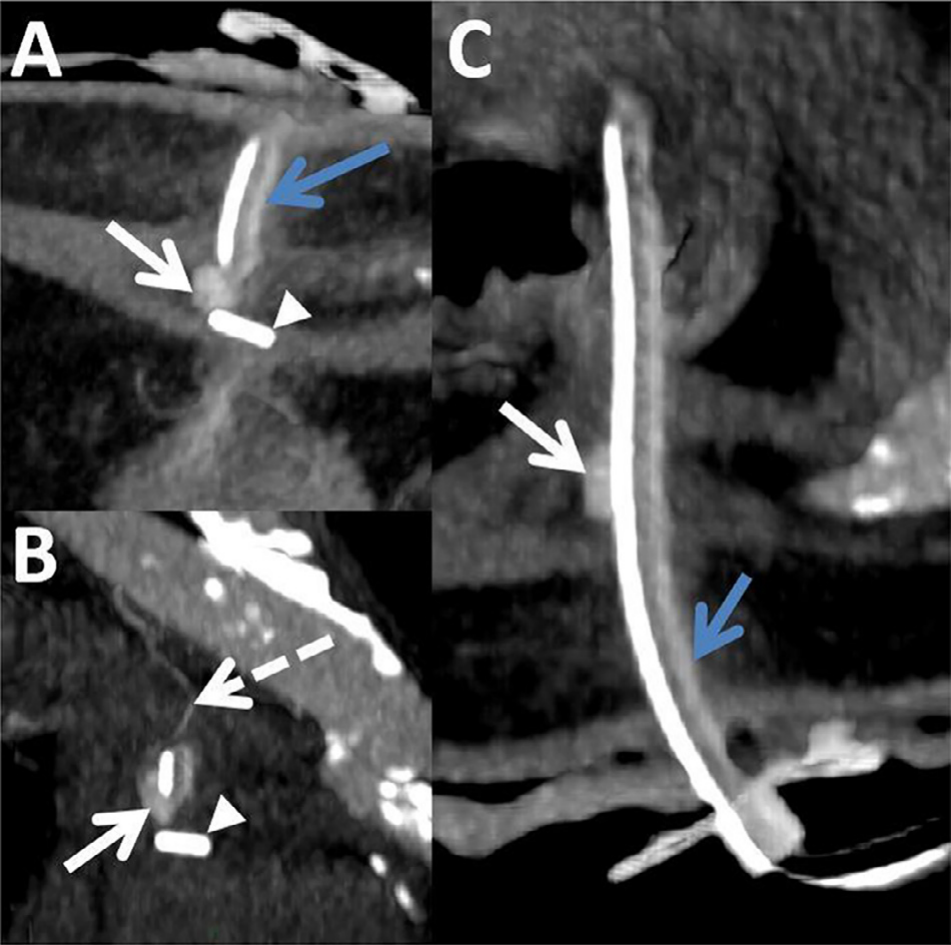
Abdominal CT scan injected at arterial time in axial (A), coronal (B) section and along the long axis of the gastrostomy tube (C), showing an addition image (white arrow) of 9mm compatible with a pseudoaneurysm, in contact with the gastrostomy tube (blue arrow) and the anchor (arrowhead), in the continuity of the upper epigastric artery (dotted arrow).
